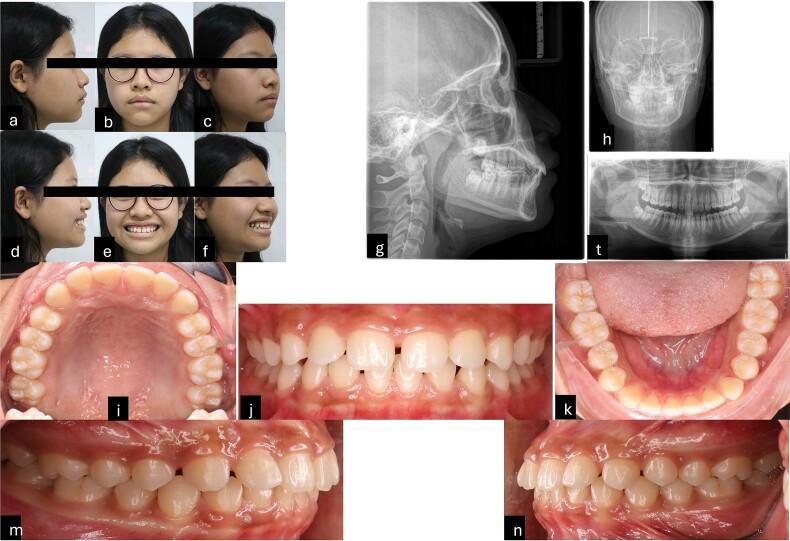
Pre-treatment records. (a–f) Extra oral pictures; (g–t) pre-treatment radiographies; (l–n) intraoral pictures.
